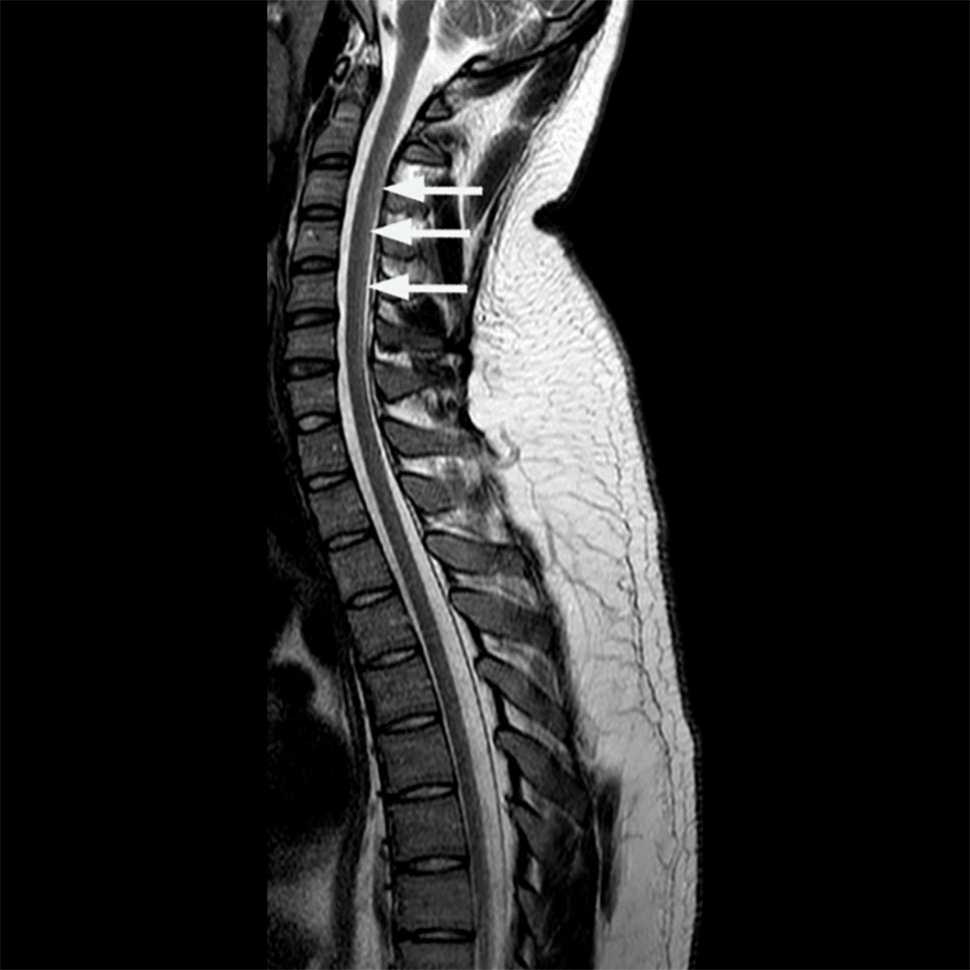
T2-weighted MRI; sagittal view of cervical and thoracic spine showing very subtle increased signal in mid and posterior cord (white arrows)
